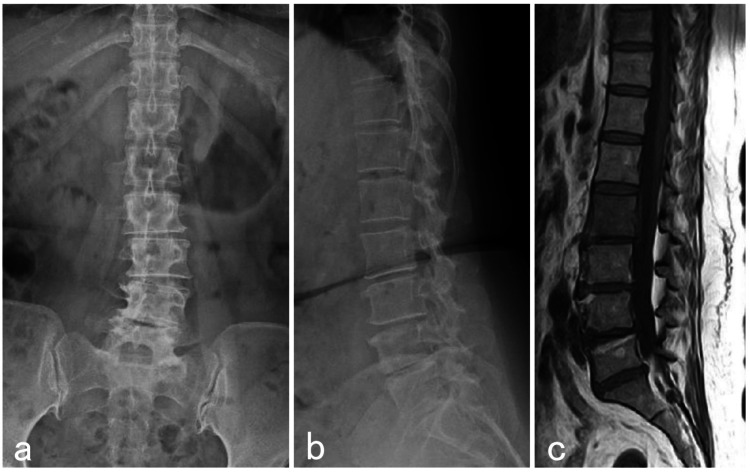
Initial imaging examination Anterior-posterior radiograph shows the lumbarization of S1 vertebrae (a). Lateral radiograph does not show vertebral fracture (b). The magnetic resonance imaging with a T1-weighted sagittal image reveals a hypointense change involving the T11 and L3 vertebral body (c).
